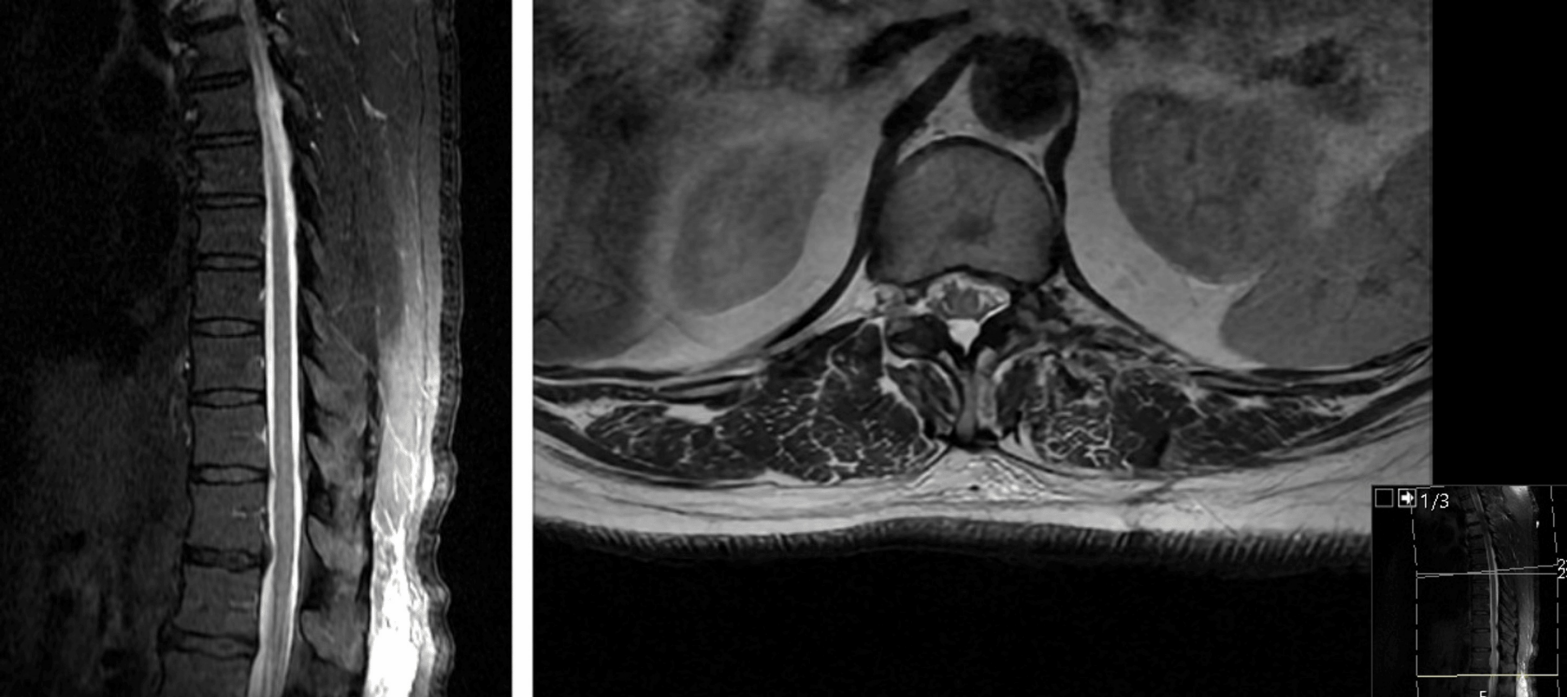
Postoperative imaging Postoperative magnetic resonance imaging (MRI) in the sagittal (A) and axial (B) planes demonstrating stable postoperative changes.
